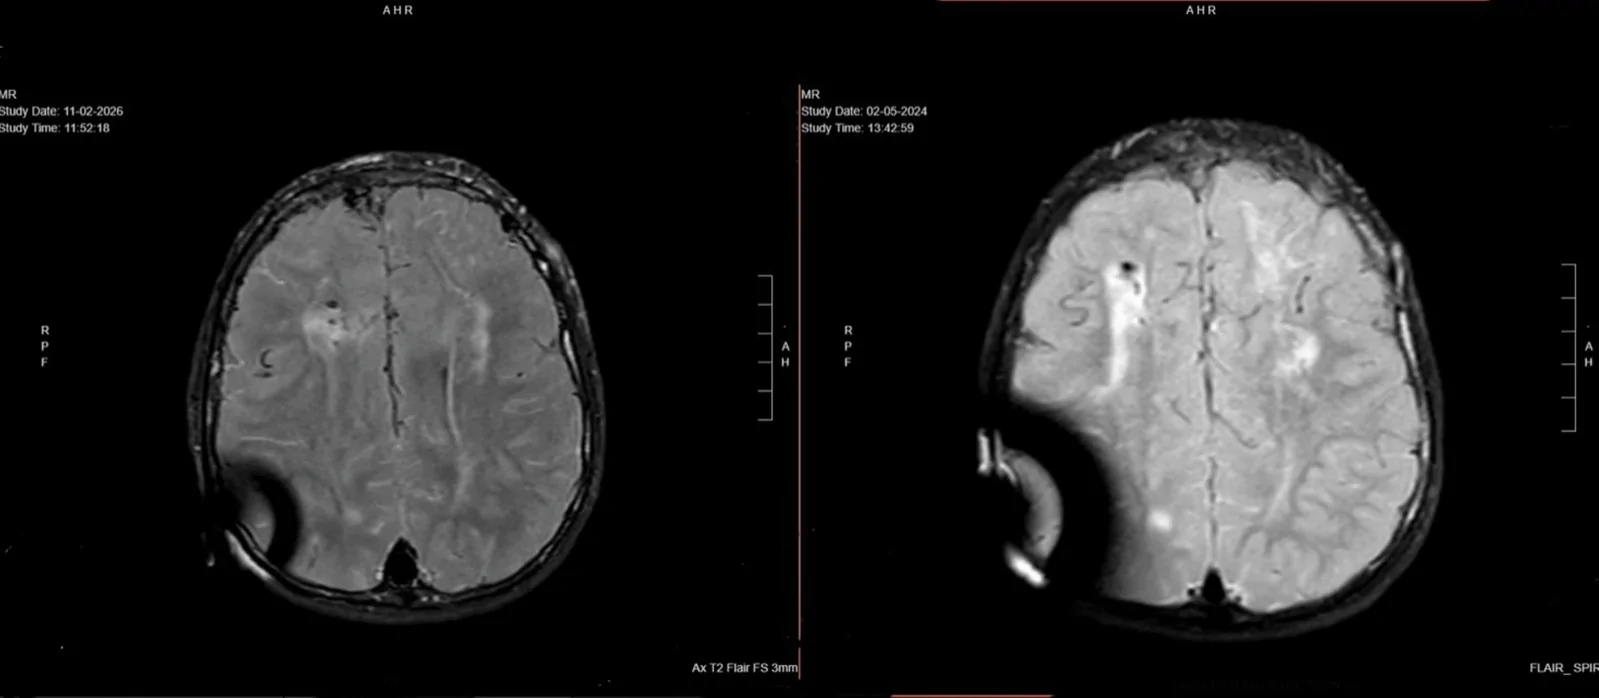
Axial T2-FLAIR MRI at the level of the lateral ventricles, comparing May 2024 (right) and February 2026 (left) studies, showing interval changes in the dural venous sinuses and pial venous structures of both frontal convexities, in keeping with recurrence of the treated vascular malformation
